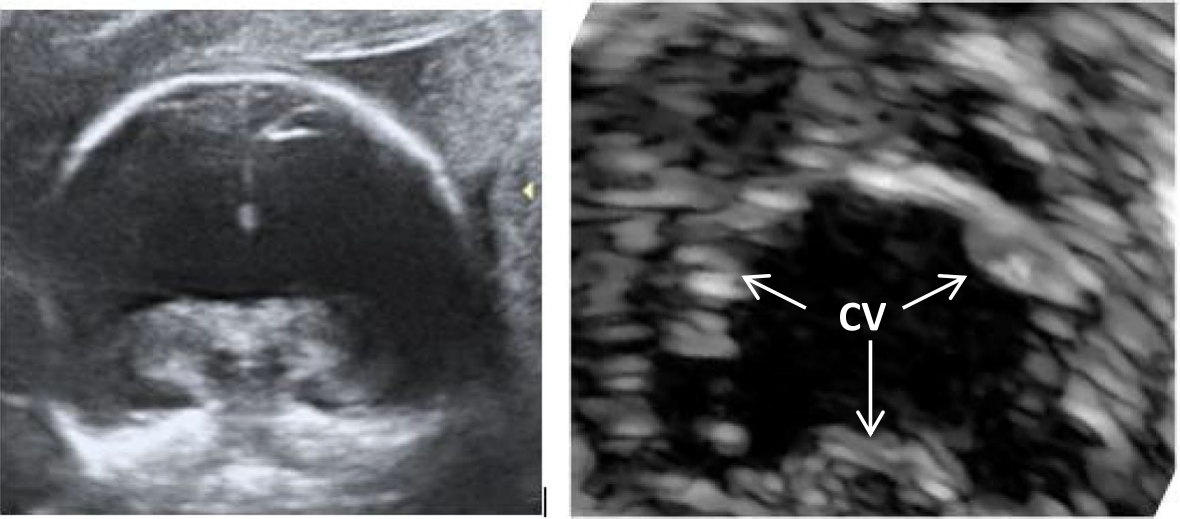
a Coronal sonogram of fetal head with alobar holoprosencephaly. b Echocardiographic subcostal view of common atrioventricular valve in the context of complete AVCD. CV: common valve
